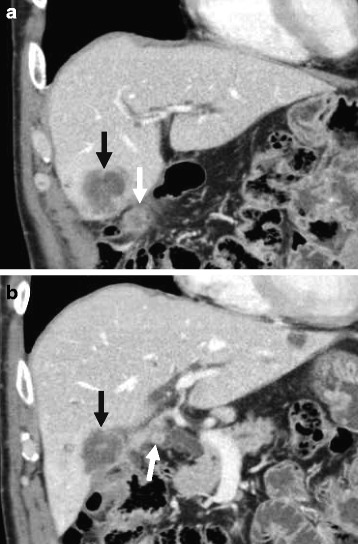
Multidetector-row computed tomography (coronal section images). a A tumour with an ill-defined border (*black arrow*) was found in the medial segment (segment 4) 1 month after the initial cholecystectomy. There was a marginal contrast effect in the mass, so the possibility of a postoperative abscess or a carcinoma that had invaded the gallbladder was considered. A mass was also found on the ventral side of the transverse colon, and there was a possibility of peritoneal dissemination (*white arrow*). b In addition, another small mass was found at the stump of the cystic duct (*white arrow*)
